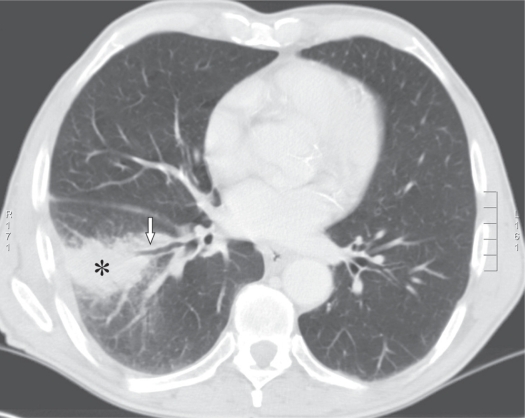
Axial CT image showing the consolidation in the right lower lobe (asterix) and the lateral basal segment of the right lower lobe bronchus (arrow).
